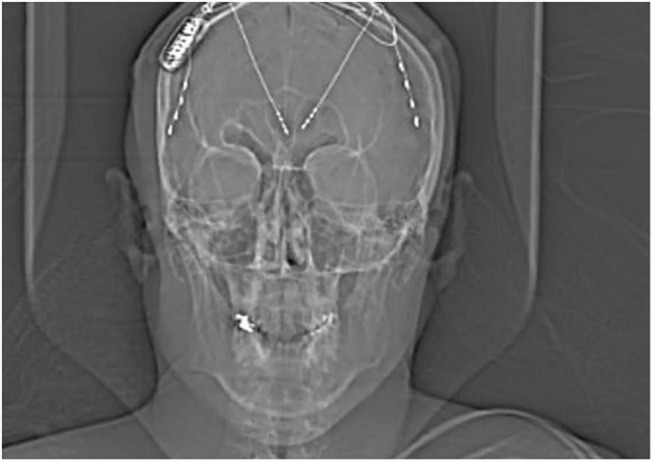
X-ray displaying the implantation of RNS device with 2-depth electrodes each with four contacts targeting the ANT and 2-strips in the prefrontal cortex of a 34-year-old patient with genetic generalized epilepsy; only the right ANT depth and right cortical strip were attached to the RNS device. Adapted with permission from Herlopian et al. (2019).
